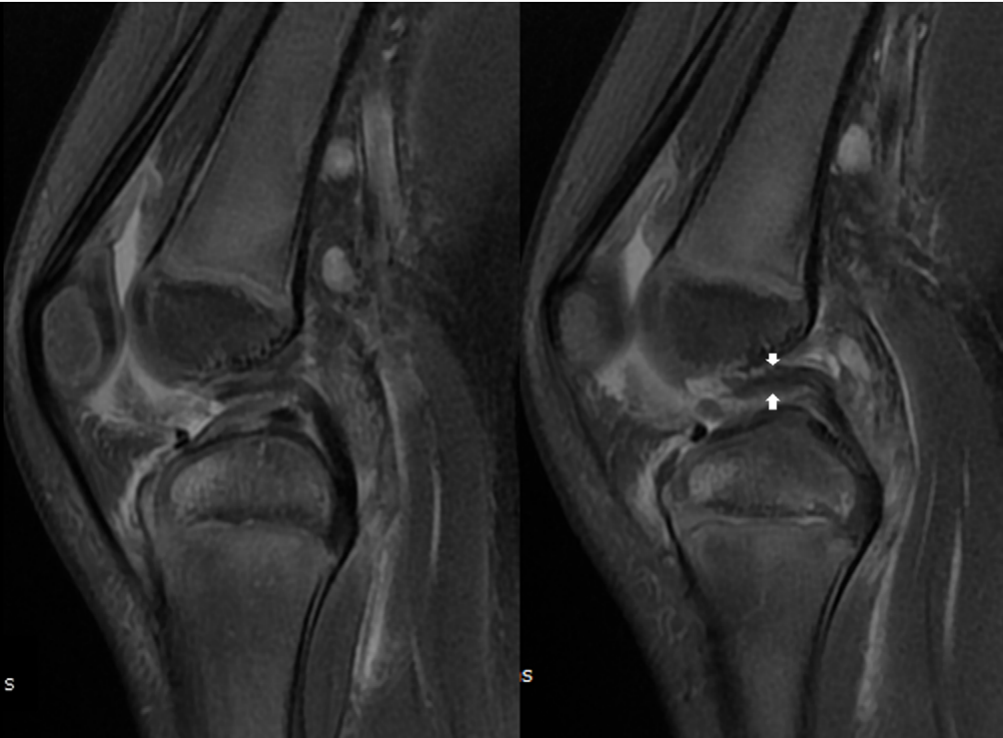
Case 2. First MRI with DP FS and sagittal sequences. Thickening of the proximal part of PCL (white arrows) with decreased PCL angle (right) and intact ACL (left).
